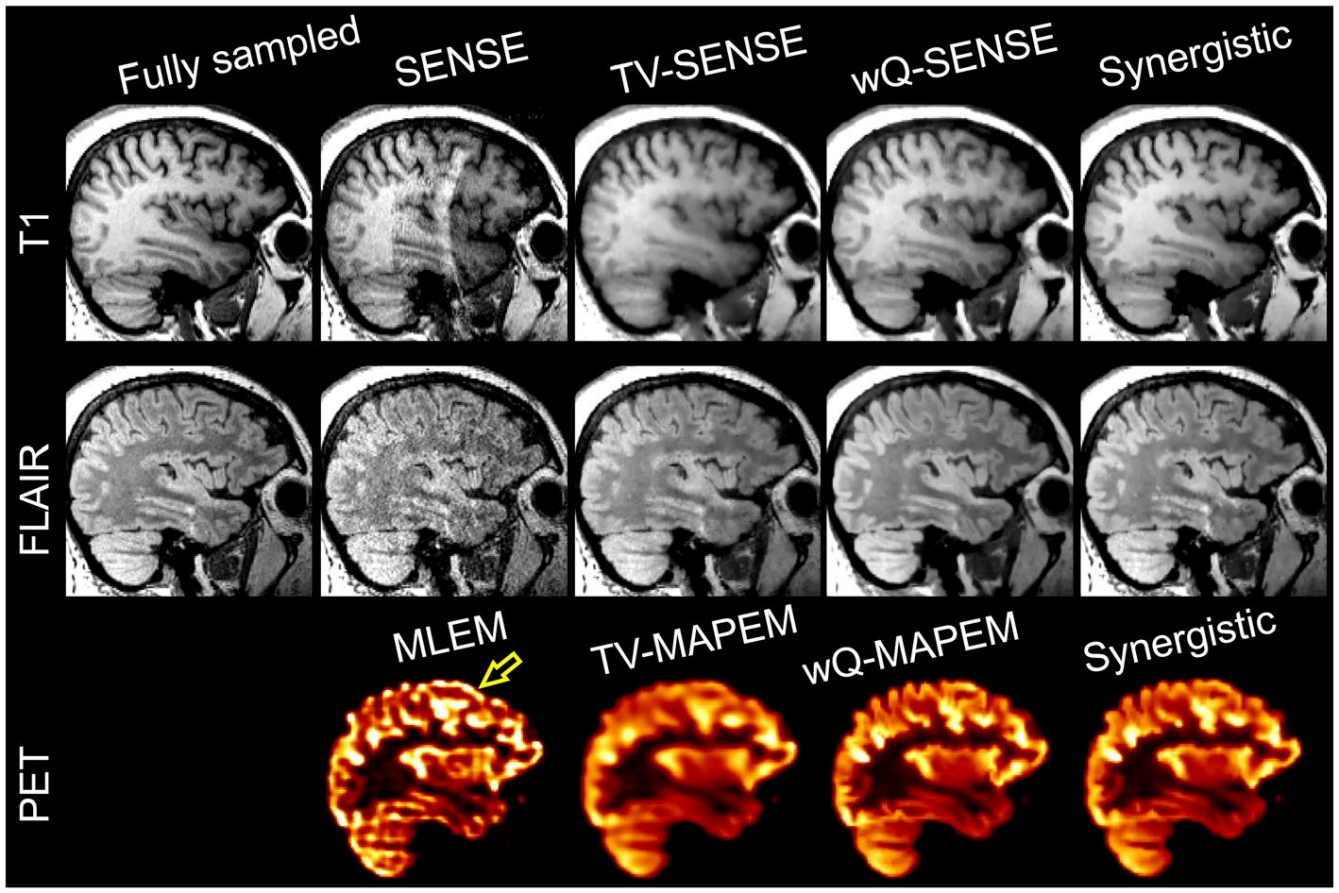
Comparison of different synergistic PET‐MR image reconstruction of the in vivo PET‐MR dataset.
